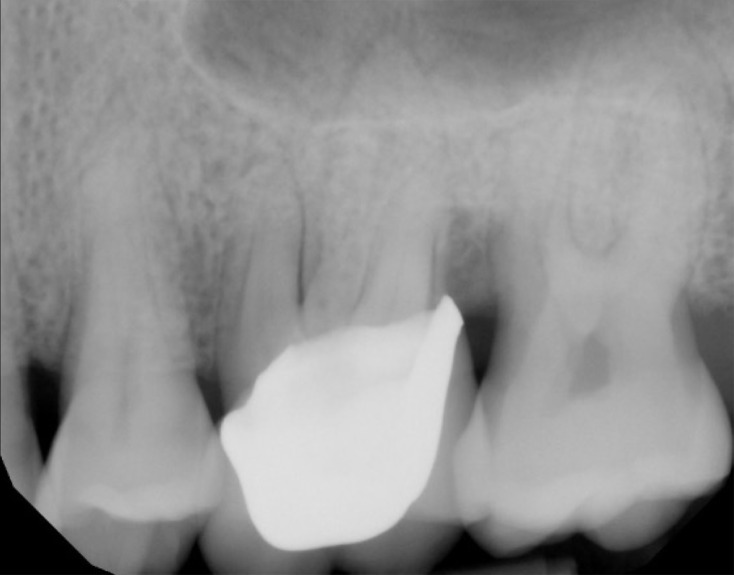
Pre-operative and 6-month post-surgery radiographs demonstrating significant bone fill at sites 25 to 27.
